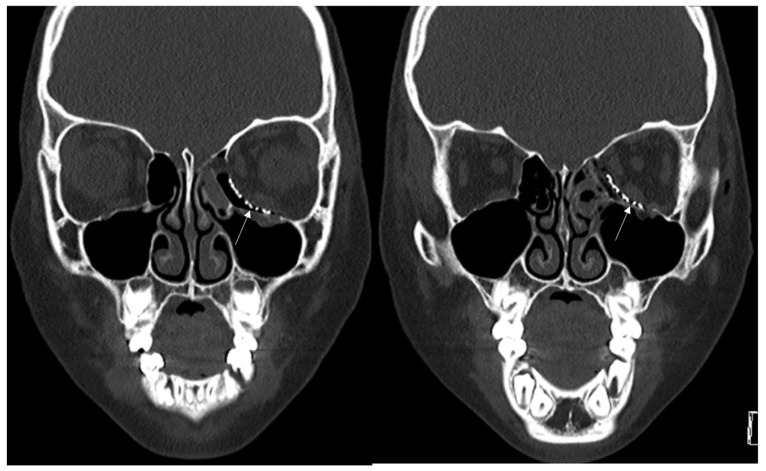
Case 1. Follow up facial CT finding (coronal view) of the same patient. The titanium-reinforced porous polyethylene (TR-PPE) plate placed on the inferomedial wall using a single screw at the inferior orbital rim. (White arrow).
